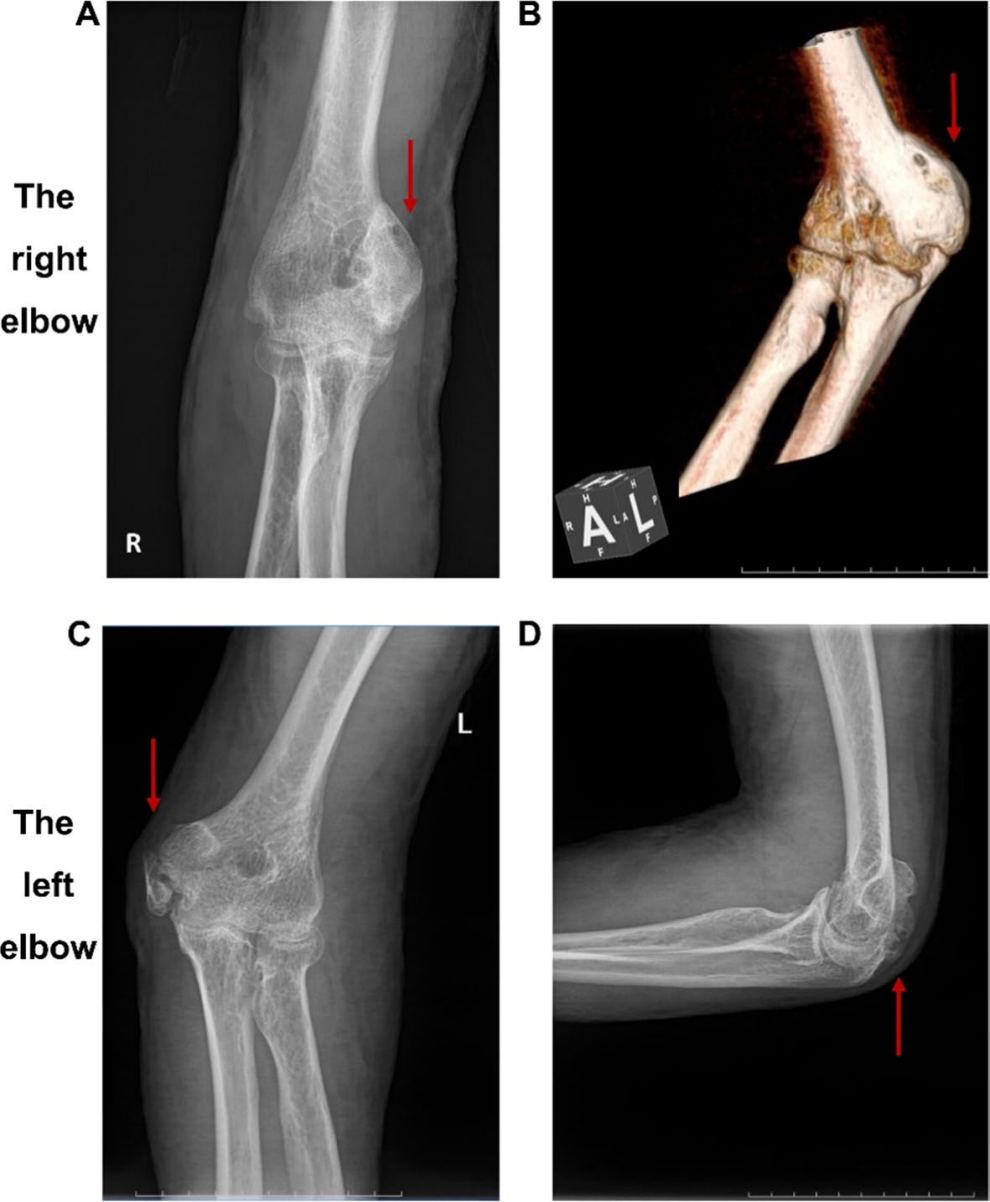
Representative imaging of burn-induced HO in the elbow. A 40-year old male patient suffered extensive thermal burn injury (80% TBSA). He complained of limitation in the range of movement of his bilateral elbow joints 3 months after the burn injury. He was diagnosed with burn-induced HO of both elbows via imaging examinations. A X-ray of the right elbow. B 3D reconstruction of CT on the right elbow. C, D X-rays of the left elbow. The red arrows indicate the HO lesions
